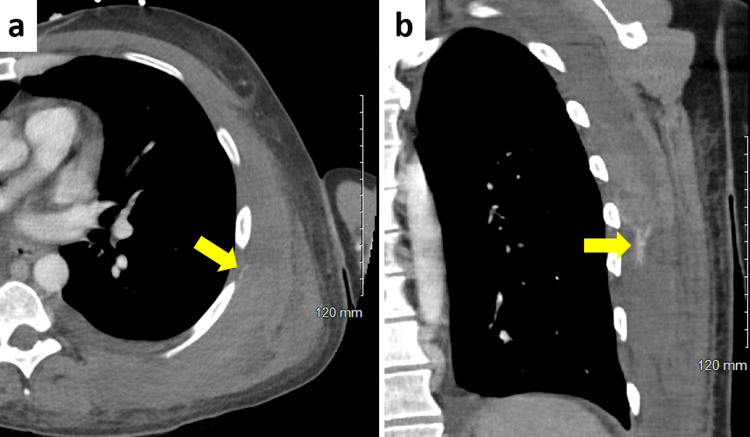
Contrast-enhanced abdominal CT scan showing contrast extravasation at the level of the sixth intercostal space (arrow): (a) axial view and (b) coronal view
